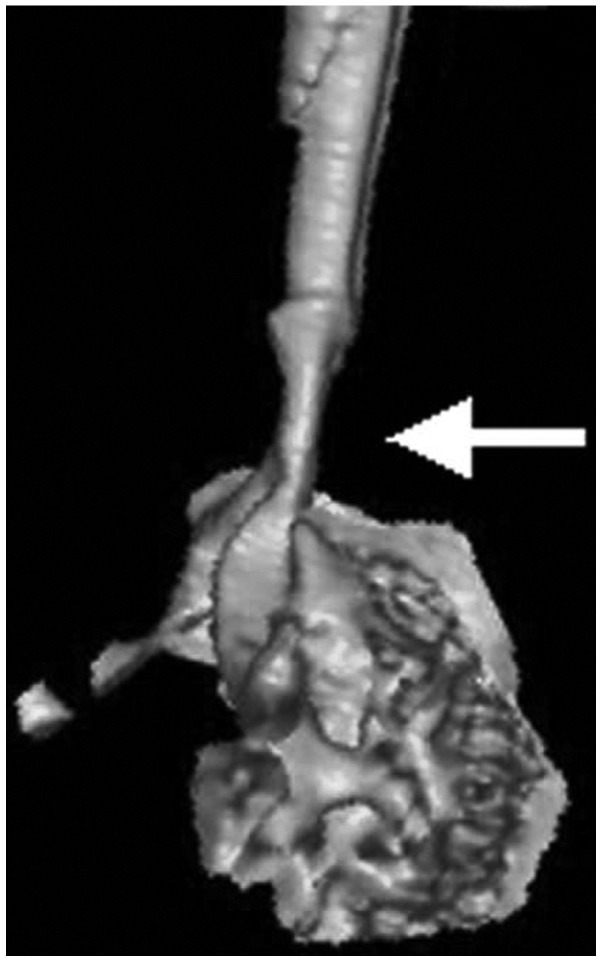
A seven-month-old male infant with DAA. The CT volume-rendered image shows lower tracheal stenosis (white arrow). DAA, double aortic arch; CT, computed tomography.
